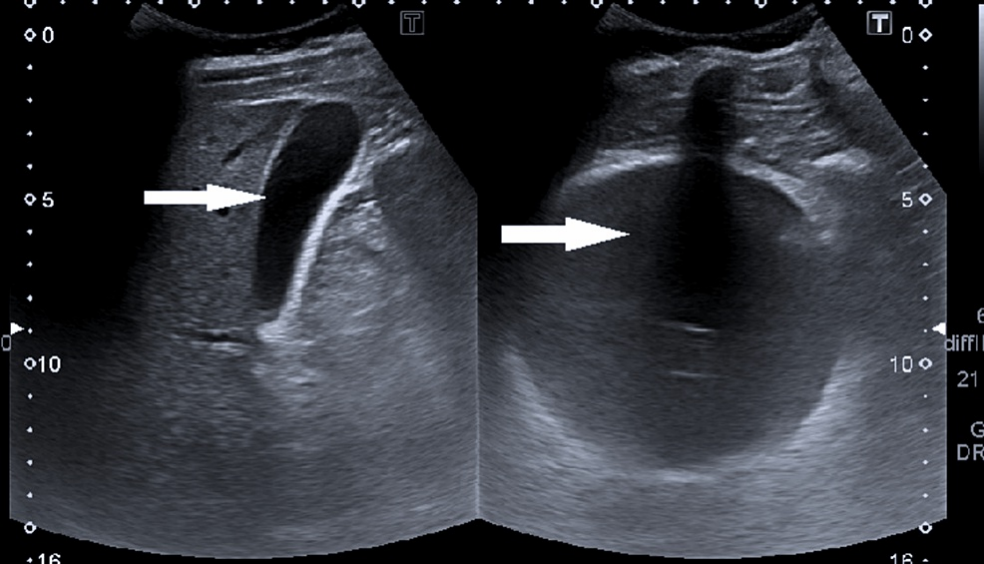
Ultrasound of the abdomen showing an intragastric balloon
